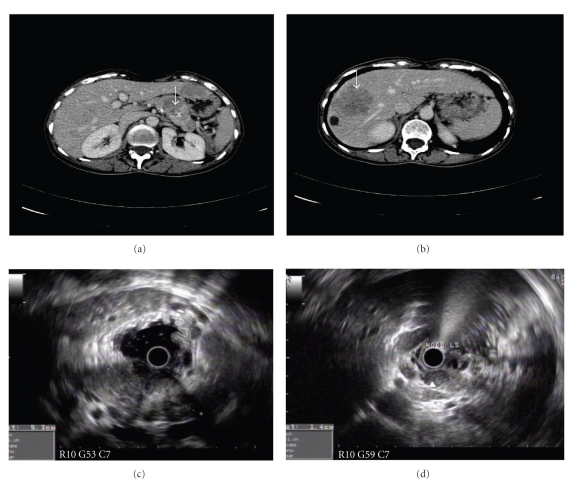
MD-CT scan demonstrating a hypodense lesion (arrow) with spots of peripheral calcifications in the body/tail of pancreas as wells as a large hypodense liver metastasis in the right lobe of liver, arrow (b). (c, d) show at endoscopic ultrasound the presence of the heterogeneous hypoechoic mass involving the body and tail of pancreas with numerous hypoechoic well defined peri-pancreatic lymph nodes (d).
